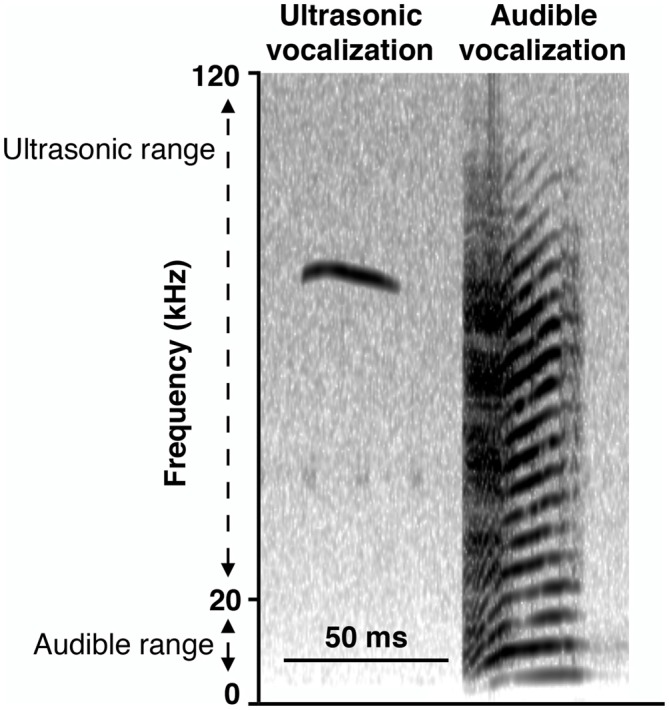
Representative spectrograms of recording of vocalizations. Ultrasonic vocalizations (USV; narrowband vocalizations in the ultrasonic range of 20–120 kHz) and audible vocalizations (AV; “squeals” with harmonics covering the larger part of the 0–120 kHz frequency spectrum, including the human audible range of 0–20 kHz) were observed regardless of when the dam was present or absent from the nest, in both control and ELS conditions.
